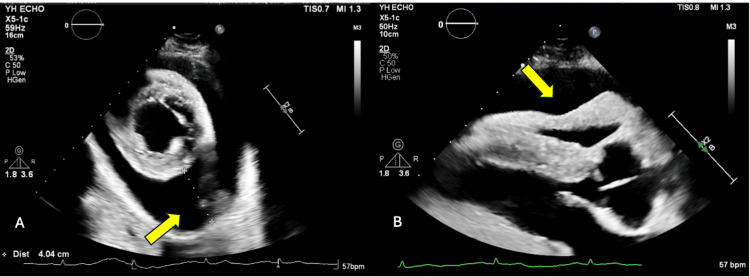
Echocardiogram views: (A) parasternal short axis (PSAX) showing a very large pericardial effusion circumferentially and (B) parasternal long axis (PLAX) showing right ventricular diastolic collapse.
